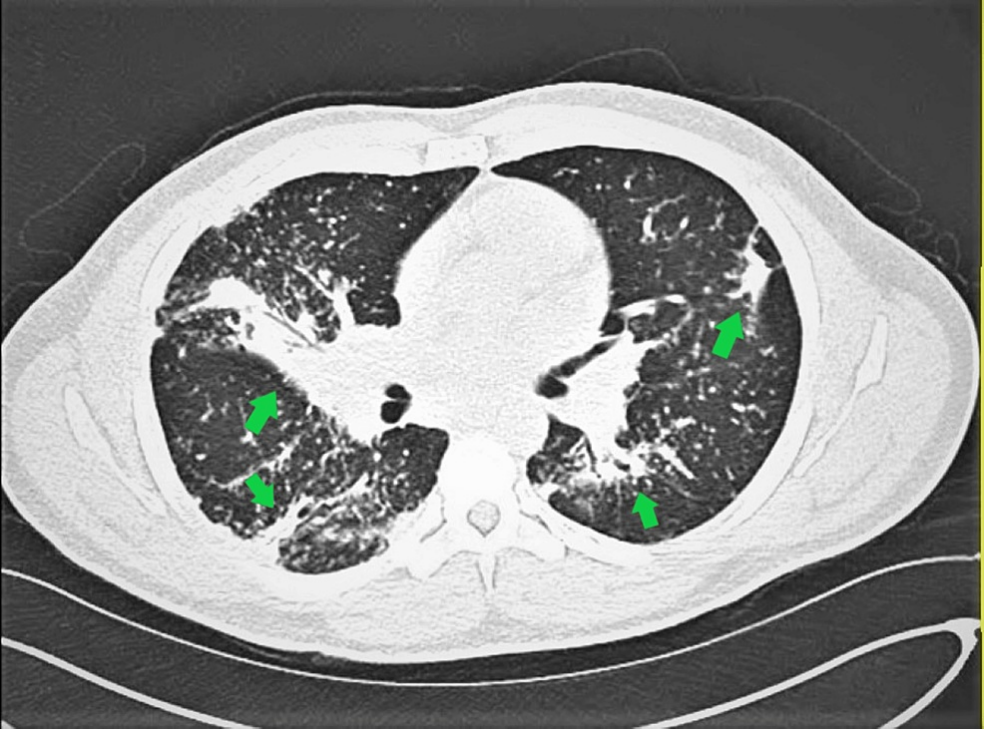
Pulmonary involvement of sarcoidosis Computed tomography without contrast showing multiple modules with more confluent opacities within the right middle and lower lobes.
